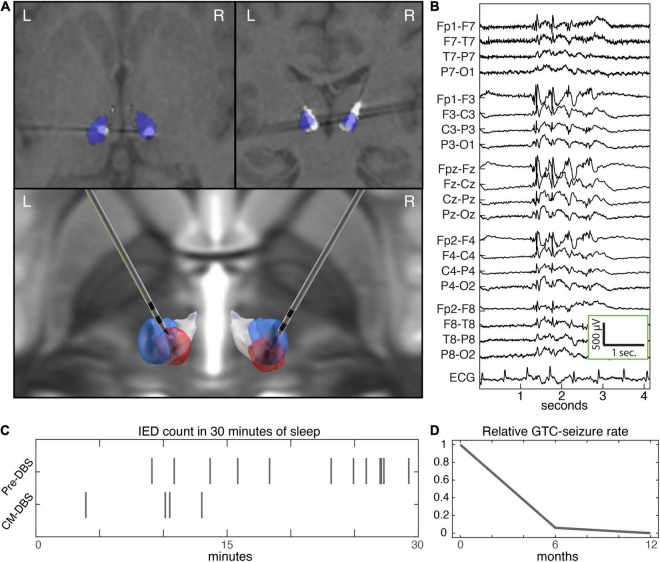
Centromedian DBS and epilepsy biomarkers. (A) Axial (top left) and coronal (top right) sections of post-operative CT coregistered to pre-operative T1 MP-RAGE MRI, and rendered implantation (bottom). The CM and Pf nuclei are colored blue and white, respectively. Neurological orientation (left hemisphere is left). The estimated VTA (red) based on stimulation parameters at last follow-up (VTA modeling with Lead-DBS SimBio/FieldTrip) (Horn and Kühn, 2015). (B) Interictal generalized spike and wave discharges. (C) Raster plot of epileptiform discharges during initial 30 min of stage N2 sleep at baseline and with CM DBS. (D) Seizure rate at baseline over the 6-months preceding DBS, and during 1 year of follow up with CM DBS. CM, centromedian; DBS, deep brain stimulation; MP-RAGE, magnetization-prepared rapid gradient echo; Pf, parafascicular; VTA, volume of tissue activated.
